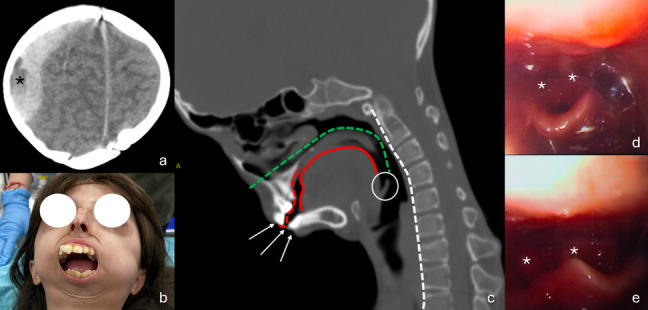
Preoperative axial noncontrast cranial CT (a), clinical photographs showing limited mouth opening and dentition (b), sagittal cervical CT (c), and endoscopic airway views (d–e). Preoperative evaluation revealed a swirl sign (a, black asterisk), a large right frontotemporoparietal epidural hematoma with midline shift, and a very low-lying glottis (c, white circle). Despite maximal oral opening (b), severe orthodontic abnormalities (b and c, red dashes), enlarged tongue volume occupying the oropharyngeal cavity (c, red curve), and conversion of ankylosis with cervical lordosis to kyphotic angulation (c, white dashes, Cobb angle 24.6°) rendered orotracheal intubation impossible (b, c). Although the glottis was visualized, passage of the intubation tube was obstructed due to glottic sclerosis, hypertrophic arytenoids (d, e, white asterisk), and limited neck mobility
